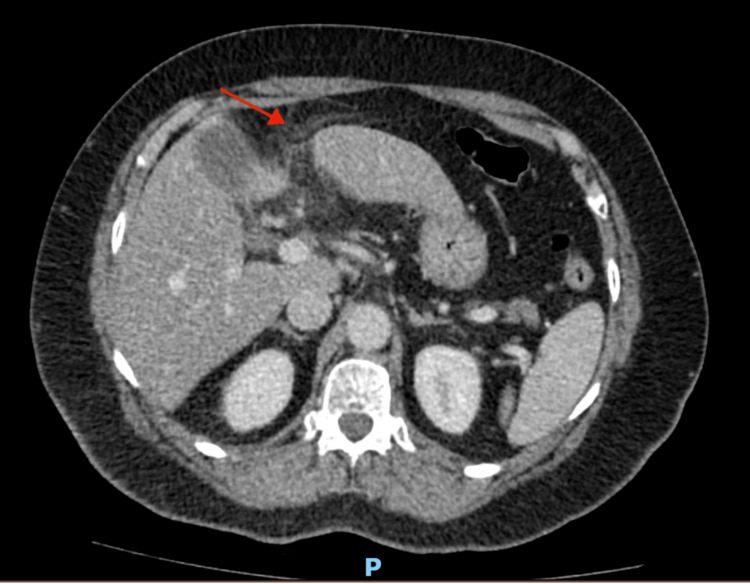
Axial view of the CT scan of the abdomen and pelvis, highlighting an inflammatory process centred on the falciform ligament.
